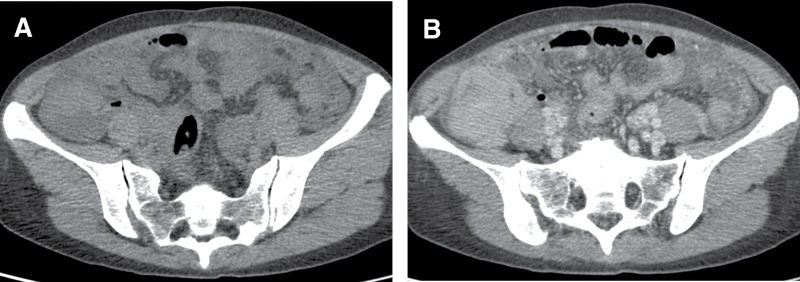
Abdominal contrast-enhanced CT showed a soft tissue mass with ill-defined boundaries in the lower ascending colon, which was significantly enhanced after contrast injection. (A) Plain scan. (B Enhancement scan. CT = computed tomography.
